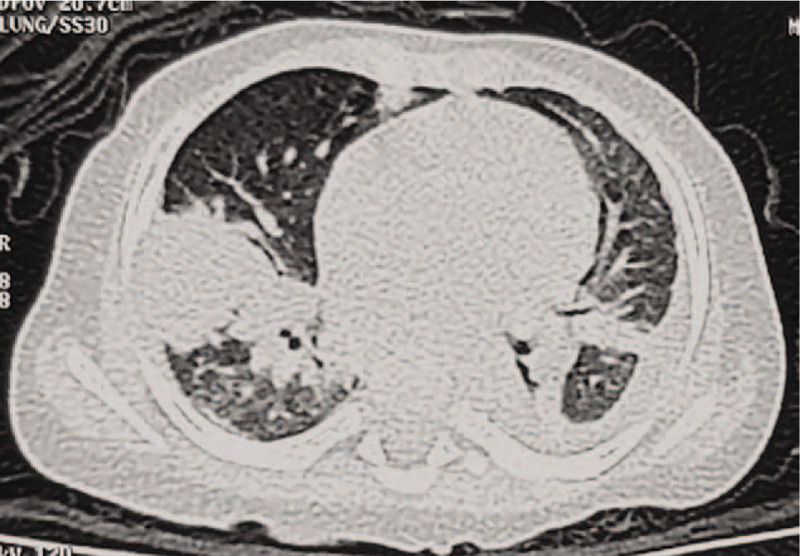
Patient #4, a boy of 2 years and 2 months who was the elder brother of patient #3, admitted to the hospital for “intermittent fever for over half a month, and cough for over 10 days.” Thoracic computed tomography 7 years after disease onset, showing multiple nodular high-density shadows or mass-like shadows.
